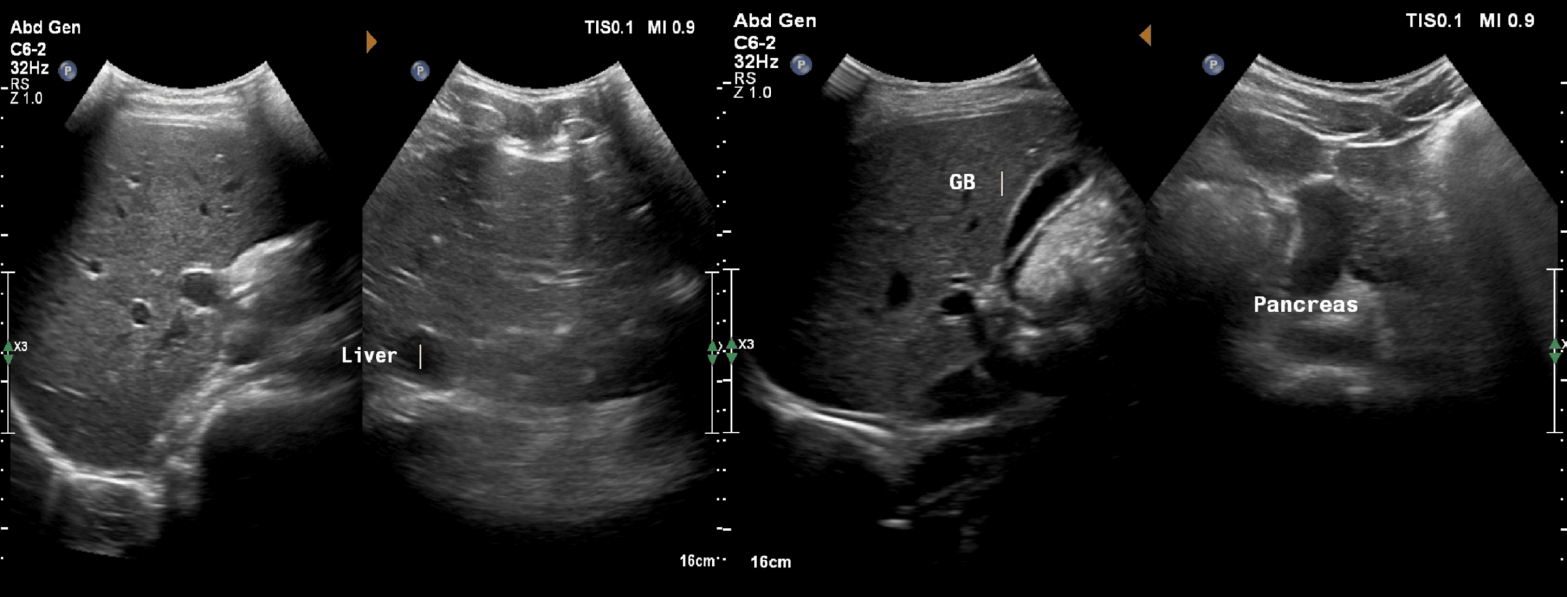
Abdominal ultrasonography was unremarkable.
